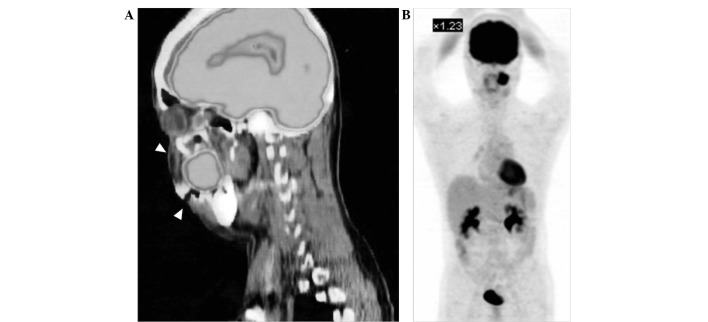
(A) Axial 18^F^-fluorodeoxyglucose positron emission tomography (FDG-PET)/computed tomography revealed FDG accumulation in the lesion in the left maxilla (maximum standardized uptake value, 12.2). (B) No other abnormal FDG accumulation was detected elsewhere by FDG-PET.
